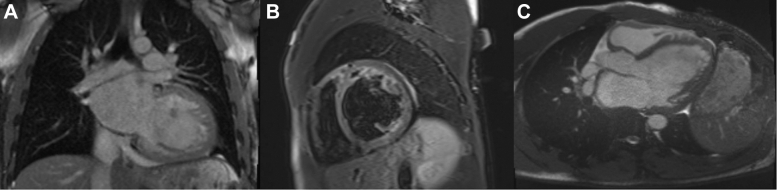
Cardiac MRI Demonstrating Sub-Acute Myocarditis With Severe Left Bi-Ventricular Dysfunction (A-C) Cardiac magnetic resonance demonstrating a markedly dilated left ventricle with severe systolic dysfunction (left ventricular ejection fraction: 12%, right ventricular ejection fraction: 12%). Left ventricular hypertrabeculation is noted, with a compacted-to-noncompacted ratio <2.3. Absence of T2 edema or late gadolinium enhancement is consistent with a subacute phase of myocarditis.
